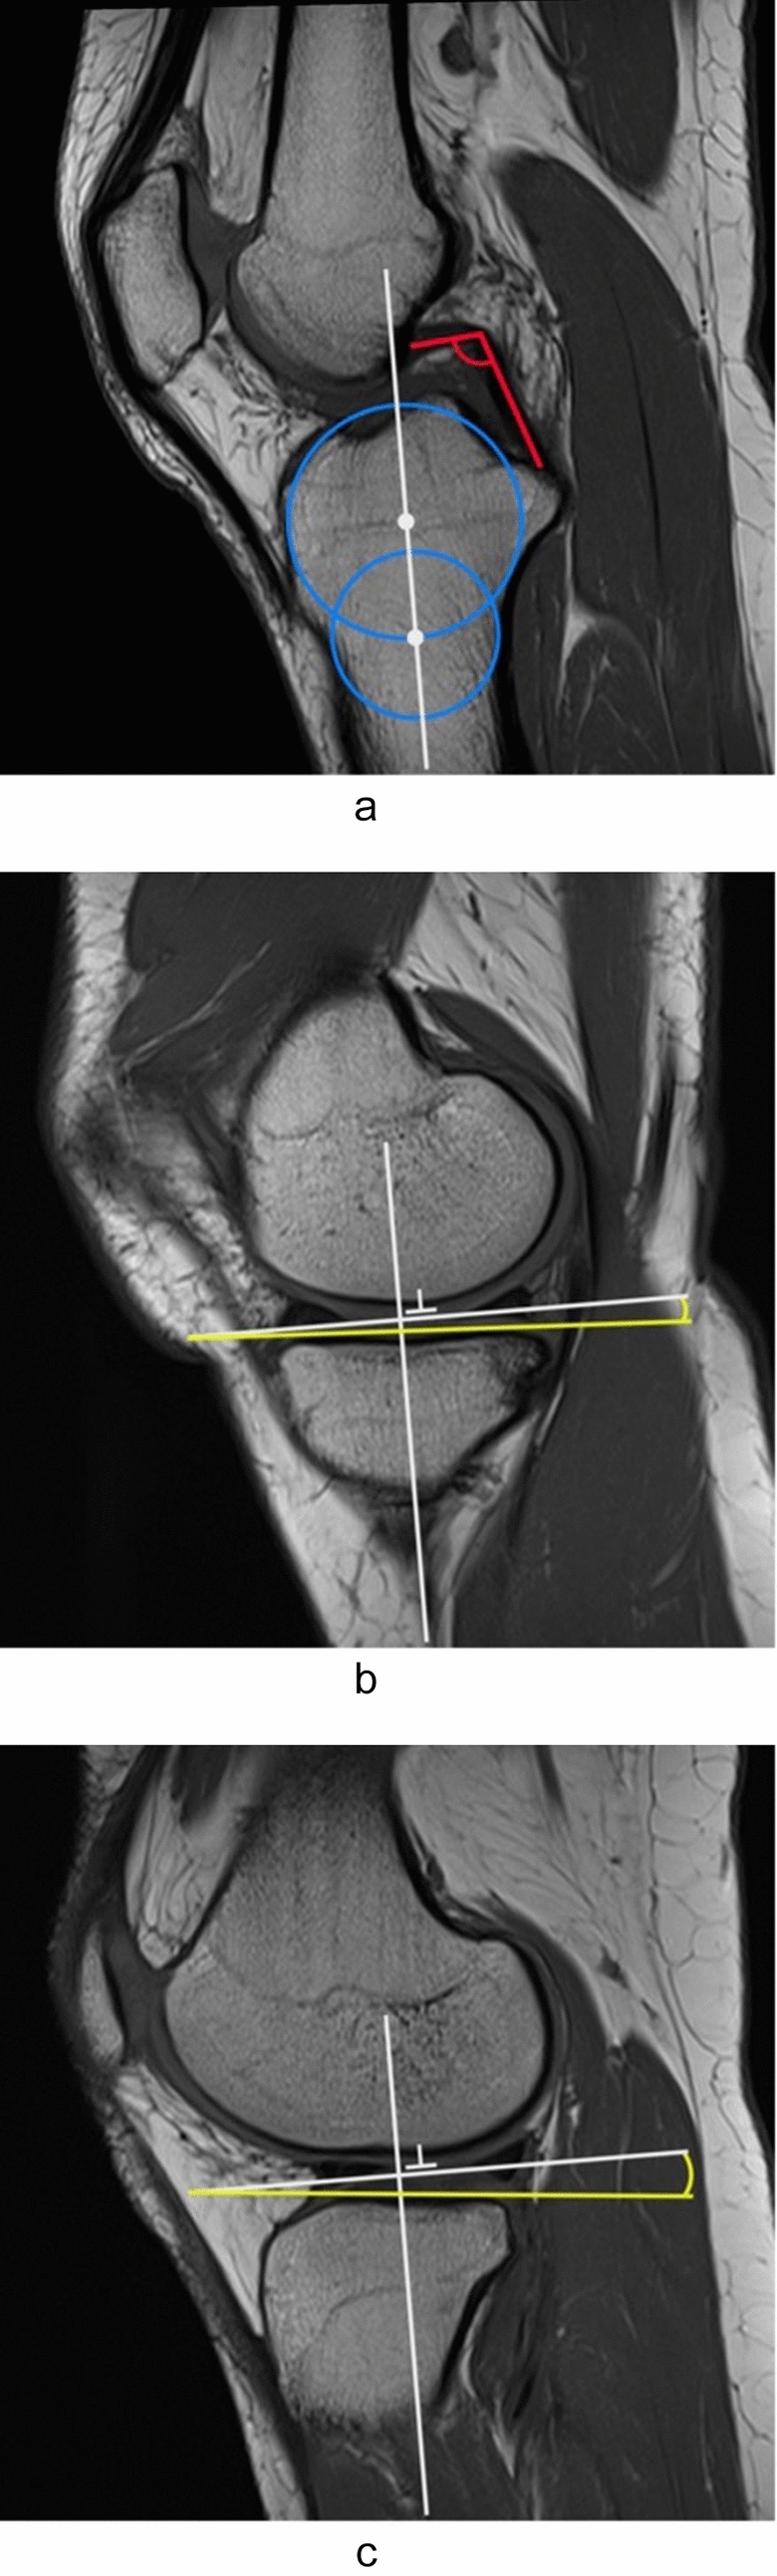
a Central sagittal slice of a T1-weighted knee MRI. The posterior cruciate ligament angle (PCLA) is formed by two lines drawn through the central portion of the tibial insertion and the femoral insertion of the posterior cruciate ligament (red lines). Blue circles represent the basis for the assessment of the tibial longitudinal axis (the white line connecting the centers of the two circles). b, c) Assessment of medial b and lateral c tibial slope. The tibial slope corresponds to the angle formed by the orthogonal line to the longitudinal axis (white horizontal line) and the tangent line (yellow line) to the center of the medial tibial b and lateral c tibial plateau
